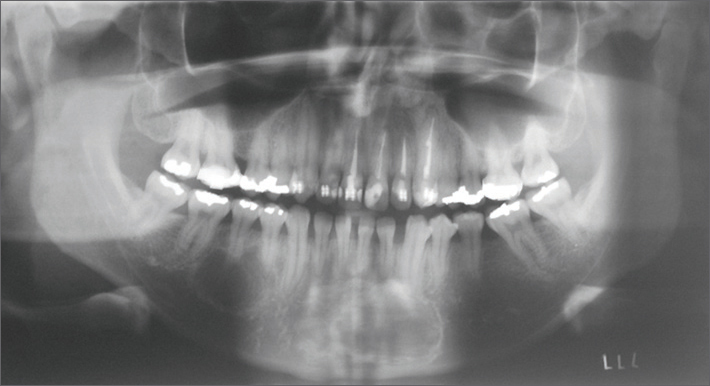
Panoramic preoperative radiograph showing radiolucent areas and projections between the apexes of adjacent teeth to the lesion in the body of the mandible.
